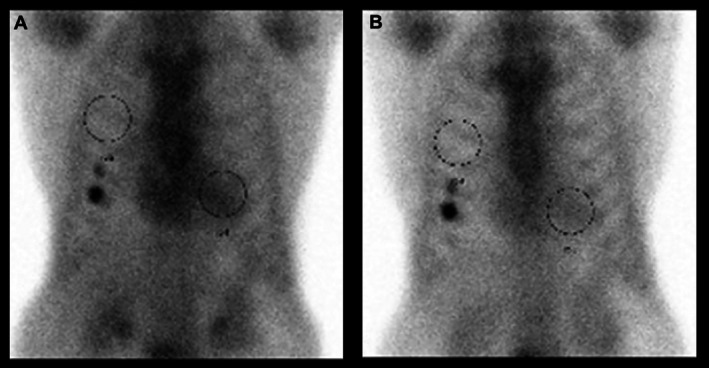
^99^ᵐTc‐pyrophosphate scintigraphy scan (A, B). A is the scan result after 1 h, and B is after 3 h. ^99^ᵐTc‐pyrophosphate scintigraphy scan showed Grade 2 cardiac uptake with heart‐to‐contralateral lung ratio of 1.54 (A) and 1.44 (B), consistent with transthyretin cardiac amyloidosis. The accumulation in the lower right rib cage is due to a past fracture.
